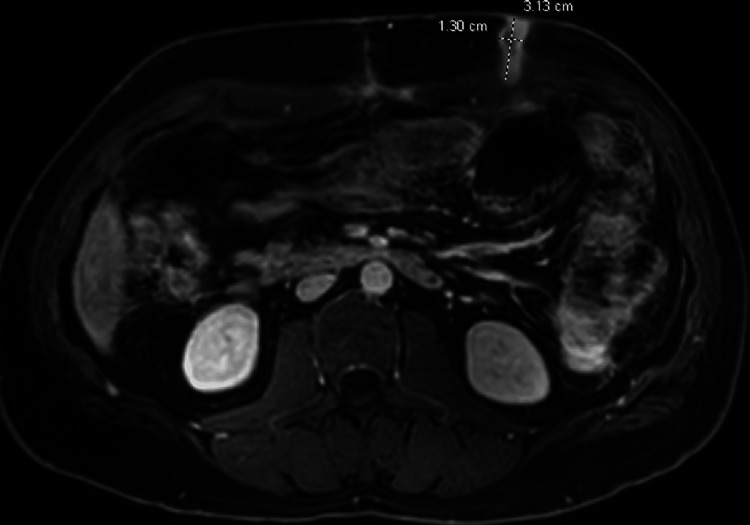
MRI abdomen with and without contrast showing enhancement of subcutaneous soft tissues around site of prior abdominal drain. Also represent normal enhancement of fibrous/scar tissue, direct seeding of cutaneous/subcutaneous site cannot be completely excluded. MRI, Magnetic resonance imaging
